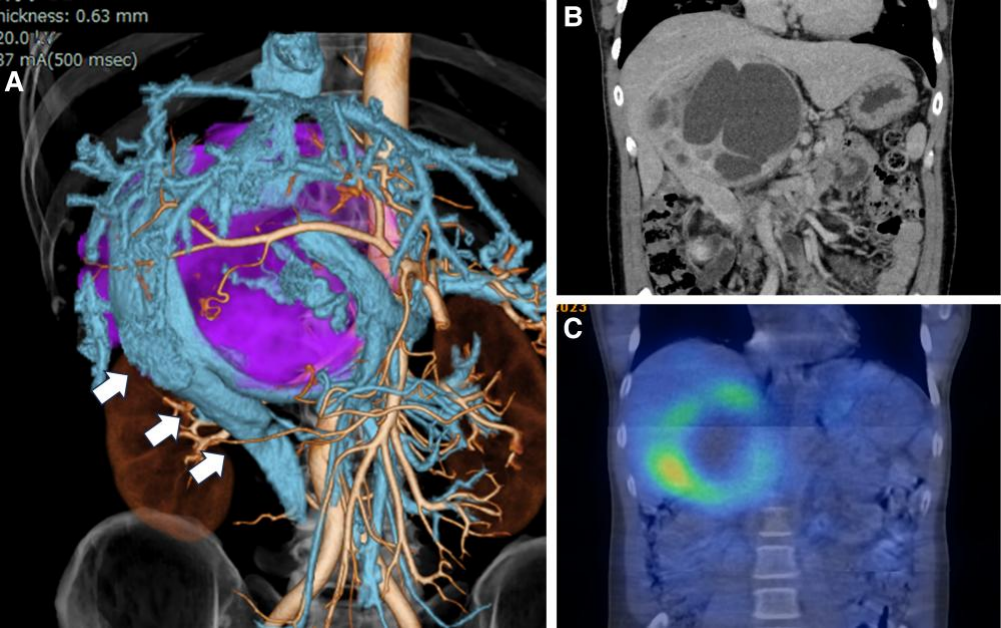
Abdominal 3-dimensional computed tomography revealing a right adrenal mass measuring 16 × 11 × 12 cm^3^ draining into the inferior vena cava, indicated by A, the white arrow. B, The tumor presents as a giant right pheochromocytoma with central necrosis. C, Scintigraphy scan with ^123^I-metaiodobenzylguanidine demonstrates uptake mainly at the tumor margins.
